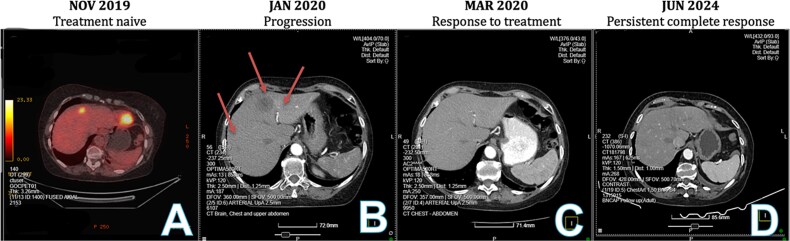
Image showing liver metastases evolution: (A) baseline imaging at the time of diagnosis from FDG-PET/CT showing two liver lesions; (B) computed tomography (CT) showing disease progression with the presence of new lesions (red arrows) from contrast-enhanced CT abdomen; (C) CT abdomen showing complete response (CR); (D) CT abdomen showing persistent CR.
